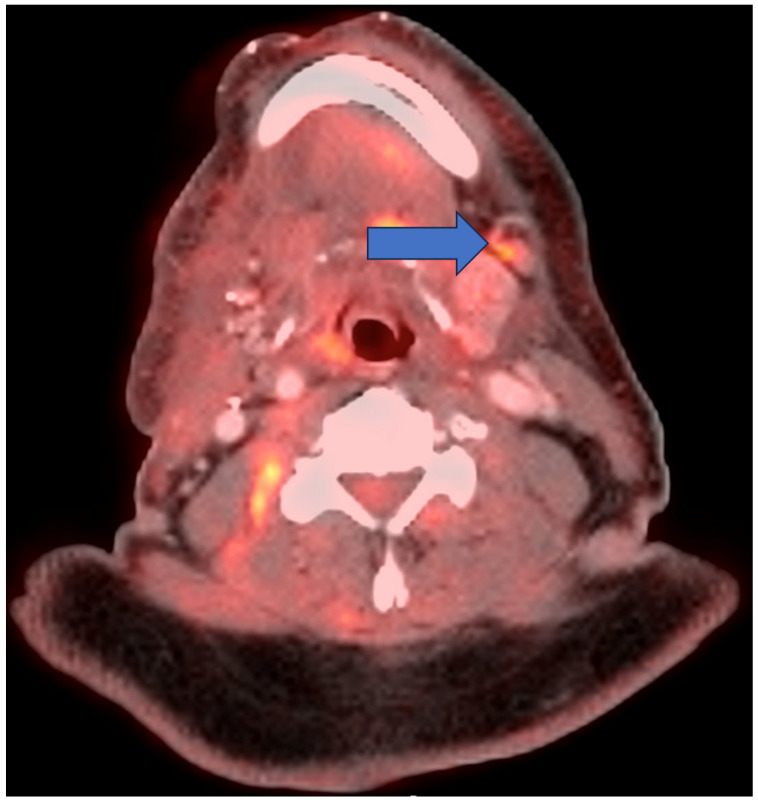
Axial fused image from a post-therapy FDG PET/CT in a 71-year-old woman with tongue cancer demonstrating a newly avid level 1B node (arrow) with uptake that is greater than in the liver and background tissues. By all criteria, this represents progressive disease. More specifically, this case is assigned PR by PERCIST given the incomplete decline; P3 (positive) by Porceddu given the uptake being greater than in the liver; H4 (positive) by Hopkins for the same reason; N3 (high suspicion) by NIRADS given the intense focal uptake; M4 (positive) by MDS for a new site of disease; and Cuneo 6 (positive) due to being greater than in the liver.
